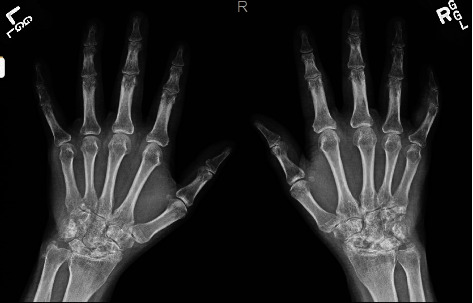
Bilateral hand X-rays with diffuse periarticular osteopenia, diffuse severe joint space narrowing involving carpel bones and radiocarpal joints, erosive changes in bilateral ulnar styloid processes, 4th and 5th carpometacarpal joints, and radiocarpal and radioulnar joints.
